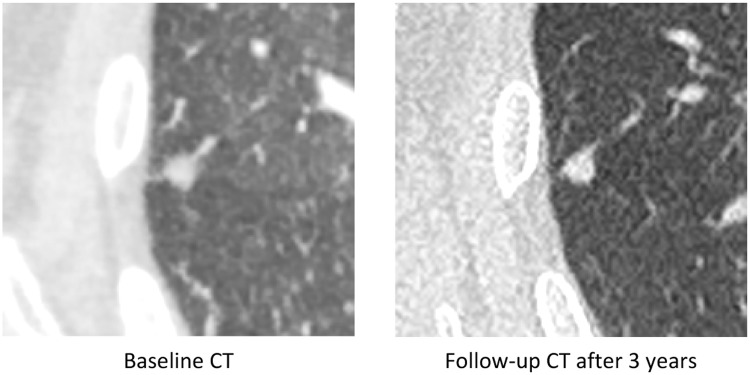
Example of a benign nodule of 8 mm of RLL in axial slices, which was subject to several follow-up CT (stability after 3 years). The nodule was already categorized as benign by the AI ​​software on the Baseline scanner (IA score <50%).
